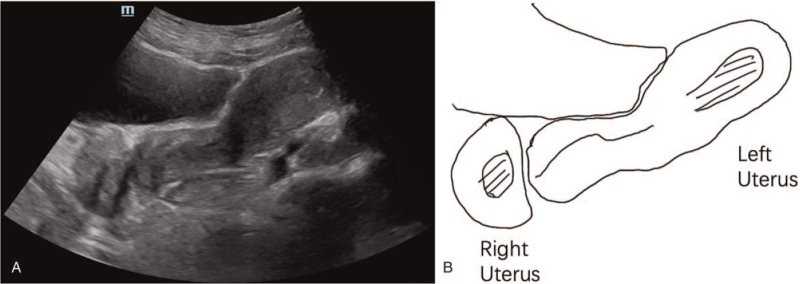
Two-dimensional transabdominal ultrasonography showing the longitudinal section of the left uterus and the transverse section of the right cervix.
